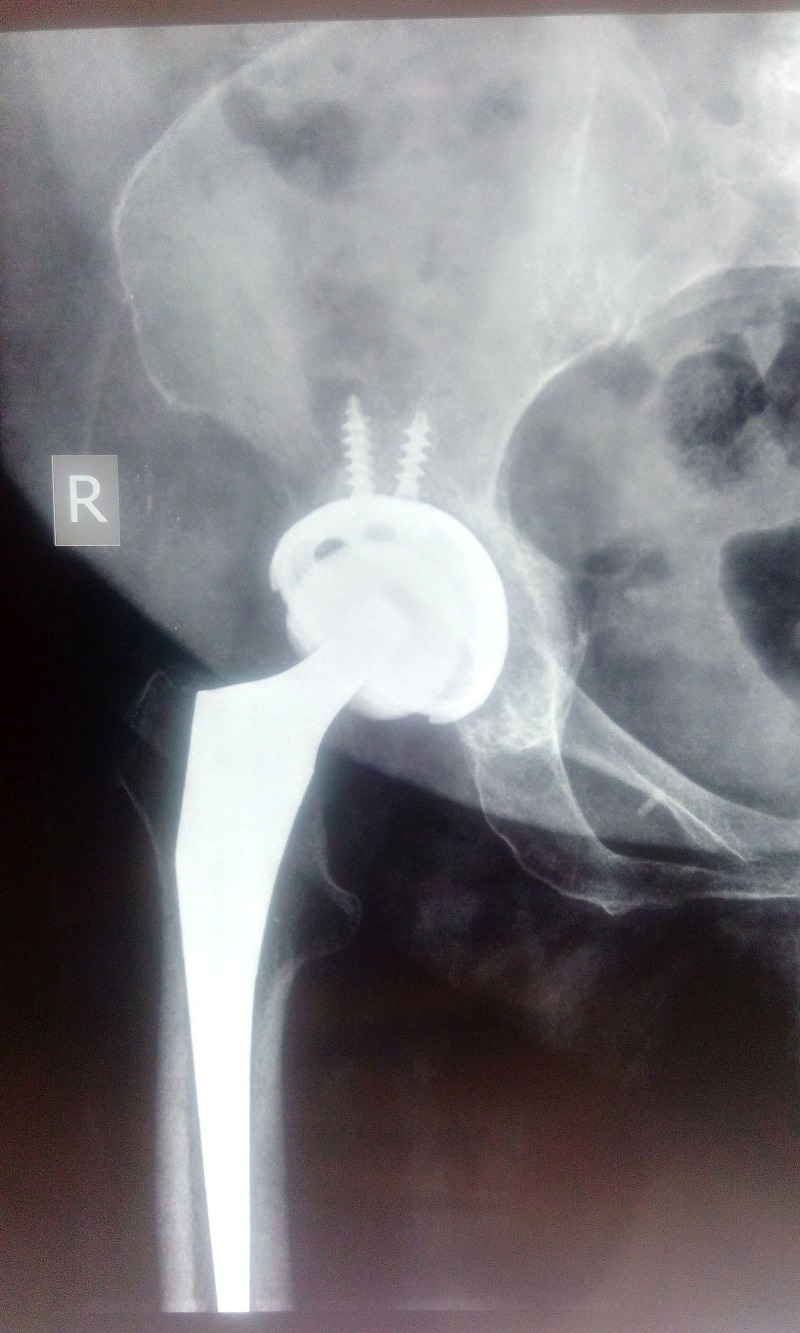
Immediate postoperative X-Ray of right hip. Anteroposterior view (AP) showing total hip replacement.
